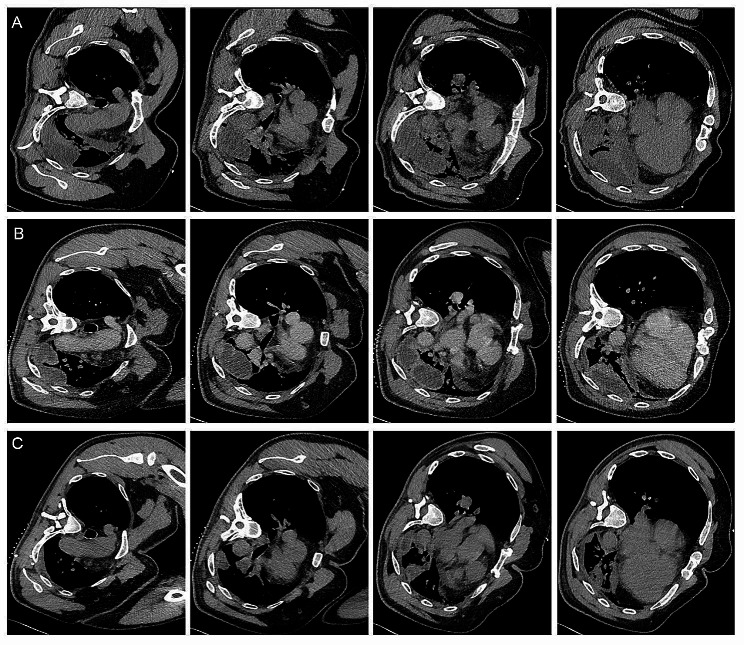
Temporal changes on chest CT imaging during the course of disease. (A) Chest CT scan before the first injection of metronidazole and sodium chloride into pleural effusion. (B) Imaging changes 7 days after the first intrathoracic injection on chest CT scan. (C) Imaging changes 14 days after the first intrathoracic injection on chest CT scan
